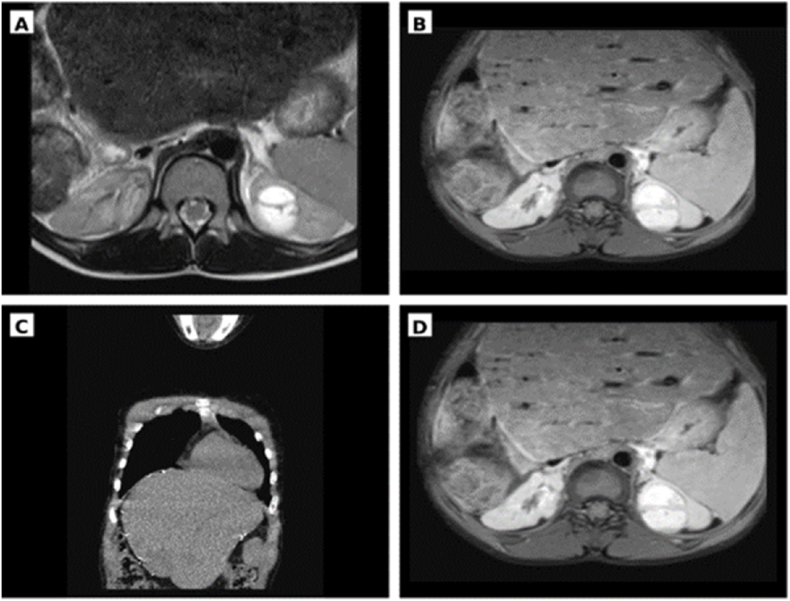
Preoperative cross-sectional imaging. (A) Axial T2-weighted MRI shows a well-circumscribed, exophytic, predominantly cystic lesion arising from the upper pole of the left kidney with internal septation. (B) Axial contrast-enhanced MRI demonstrates complex cystic morphology with enhancing septal components. (C) Coronal CT provides an overview of the upper abdominal postoperative/transplant-related anatomy. (D) Axial contrast-enhanced MRI demonstrates enhancing solid components within the cystic lesion.
